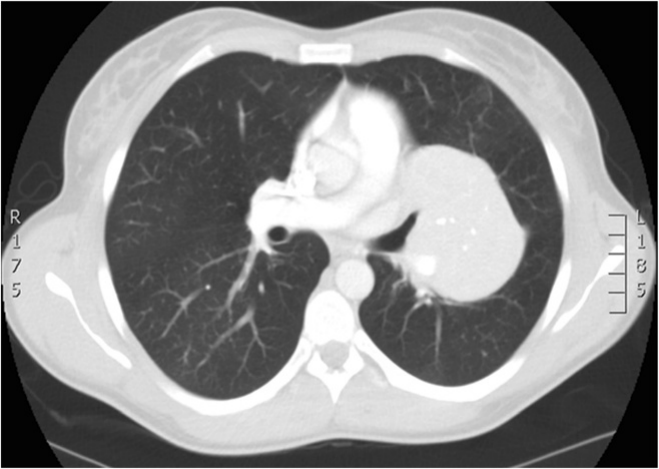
CT scan of the Castleman’s disease.
